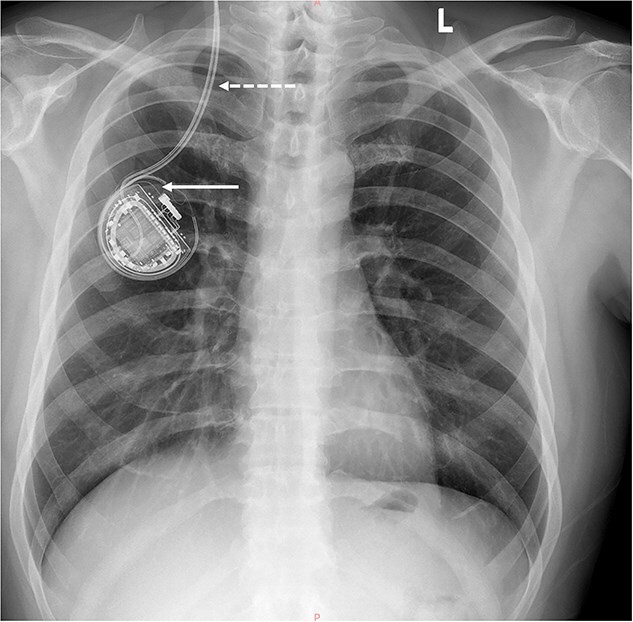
Chest radiograph obtained at the current admission demonstrating horizontal rotation of the r-IPG within the subcutaneous right chest pocket. The device has undergone approximately 180° of horizontal rotation, with the coil-receiving surface directed posteriorly away from the skin rather than anteriorly towards it, placing the charging coil in direct opposition to the skin and disrupting effective transcutaneous energy transfer. The leads exit the device from the right side (white solid arrow), with no evidence of lead coiling or displacement (white dashed arrow).
